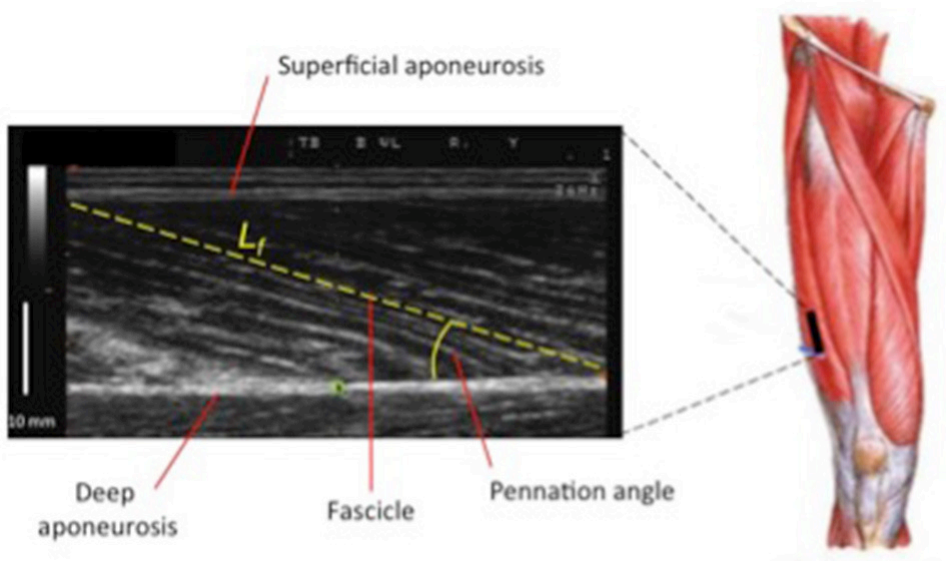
Ultrasound scan of VL muscle: Lf (fascicle length) and PA (pennation angle) represent two of the major features of muscle architecture.
